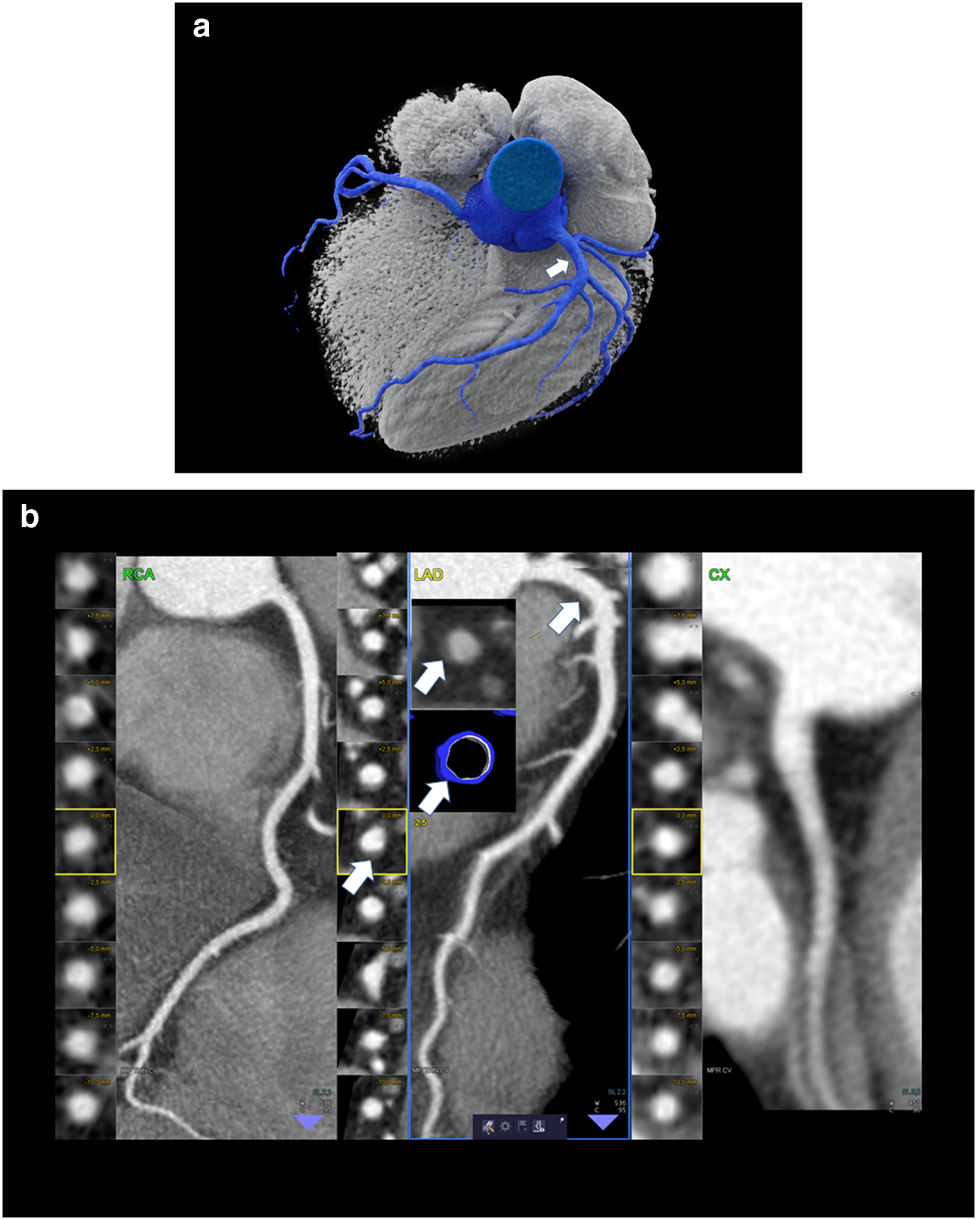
A 64-year-old male with one risk factor (arterial hypertension) and ESLD due to alcohol-related liver disease. CTA shows a small non-calcified plaque in the proximal LAD (arrow) with a high plaque density (HU 80–90) and minimal stenosis (CAD-RADS 1). Calcium score was zero. VRT (left) and cMRP (right) of the RCA; LAD and CX
